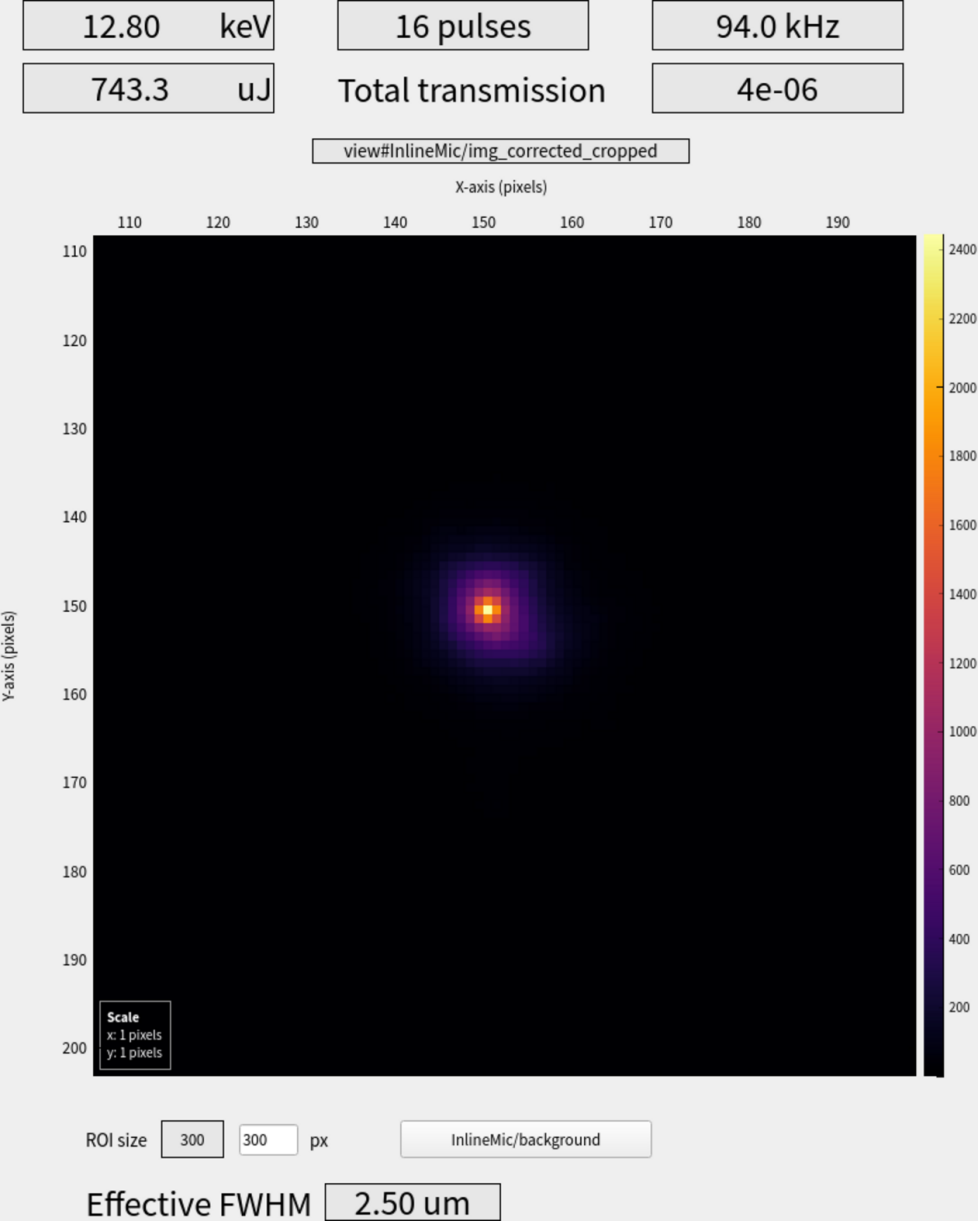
Image from *Karabo* focusing scene used to validate and optimize focus during alignment at the start of each user shift. The focal spot is only a few pixels with 1.042 µm per pixel. The measured effective FWHM (provided in *Karabo* to enable optimization) of the IRD focal spot is routinely ∼2.50 µm (in this case with 12.8 keV X-ray energy) using the direct beam trajectory. A similar effective FWHM is also achievable in the IRD for the refocused micrometre KB trajectory.
